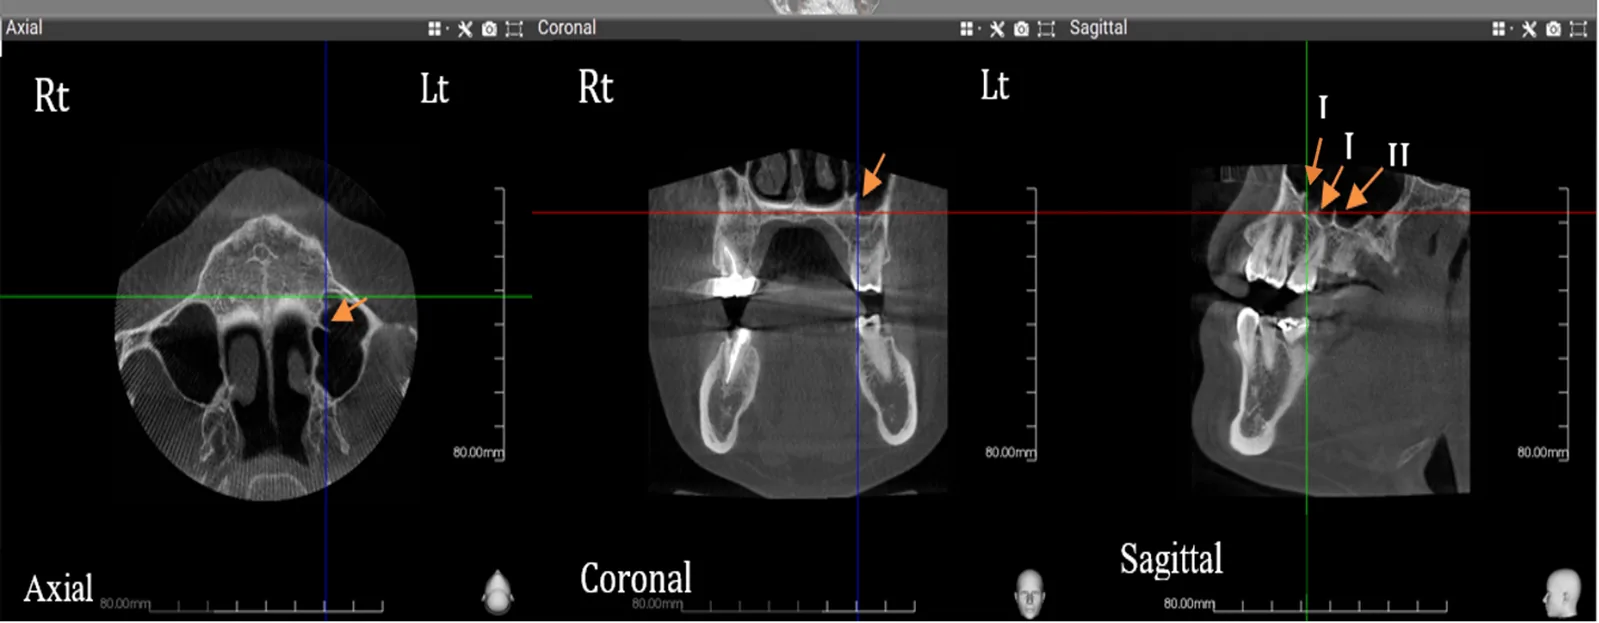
Positions of maxillary sinus septa in MPR (axial, coronal, and sagittal views) (I, Anterior; II, Middle; III, Posterior). Multiplanar reconstruction (MPR) CBCT images (axial, coronal, and sagittal views) demonstrating the location of maxillary sinus septa. Septa are classified based on their position as (I) anterior (mesial to the second premolar), (II) middle (between the second premolar and second molar), and (III) posterior (distal to the second molar). Arrows indicate the identified septa in each plane.
